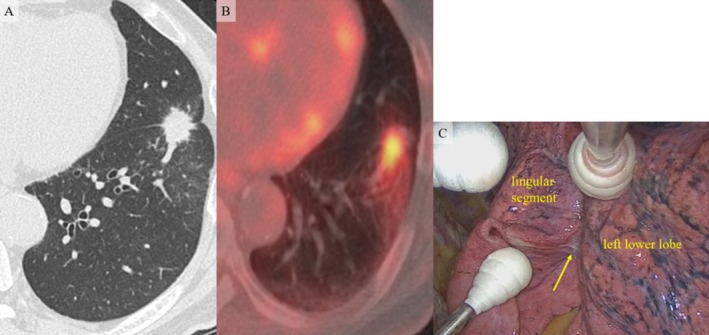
Radiological images and intraoperative findings. (A) Chest computed tomography revealed a 3.0 cm mass with spicula in the left S8 near the interlobar fissure. The mass appeared to invade the lingular segment. (B) The maximal standard uptake value (SUVmax) of ^18^F‐fluorodeoxyglucose positron emission tomography (FDG‐PET) of the mass was 4.49 on the early scan and 5.18 on the delayed scan. (C) Adhesion was revealed between the left lower lobe and the lingular segment. An abscess‐like mass (arrow) was found in the adhered interlobar space.
